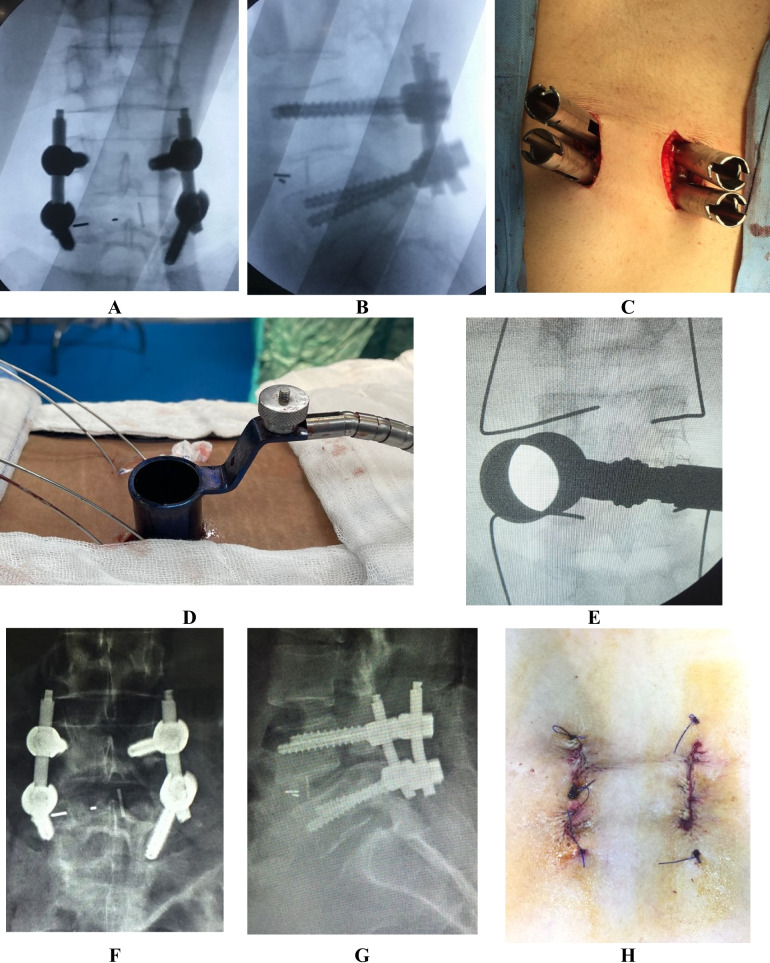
MISTLIF case: (A–B) show intraoperative fluoroscopic image antro-posterior and lateral view of the slipped level after insertion of the screws and rods, (C) show intraoperative photo of the wound, and (D) the tubular system in place, (E) C-Arm photo of the tubular system fixed on the facet (F-G) postoperative X-ray intro-posterior and lateral view of the slipped level and (H) shows the wound photo after closure of the wound
